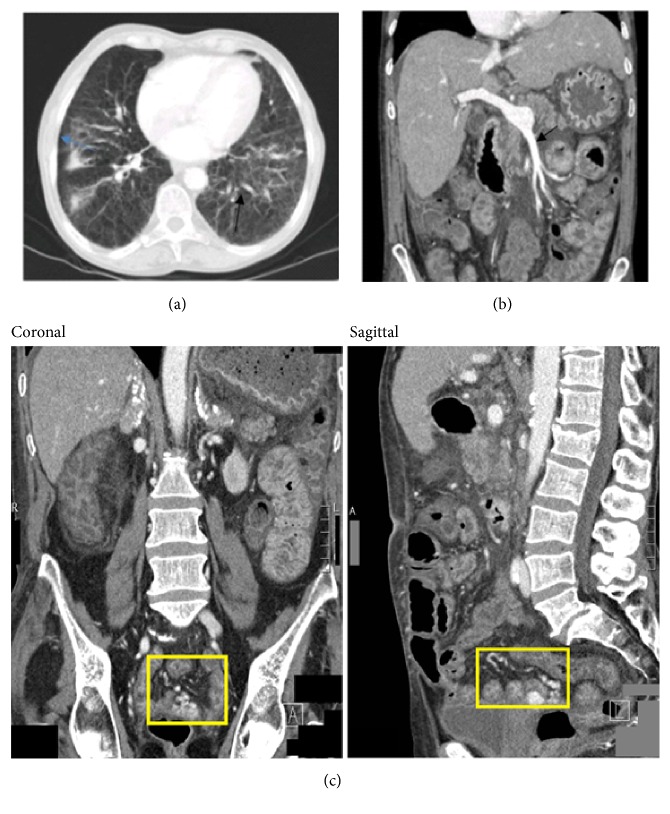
CT of chest (a), abdomen (b), and abdomen/pelvis (c)*. (a) Chest CT showing centrilobular emphysema and signs of interstitial lung disease. There are bilateral basal inter- and intralobular septal enhancement (blue arrow) consistent with sphingomyelin deposition seen in ASMD patients. Multiple bronchiectasis (black arrow) is also present. (b) Venous phase of an abdominal contrast CT, showing an enlarged portal vein (arrow) and a moderate amount of ascites fluid in the abdominal cavity. The liver is nodular, enlarged, and steatotic. The patient is asplenic. (c) Coronal and sagittal CT images of abdomen and pelvis show the cluster of varicose veins that are contrast-enhanced (outlined by yellow boxes), situated just above the vaginal lumen, and show extravasation of contrast into the small intestinal lumen.
